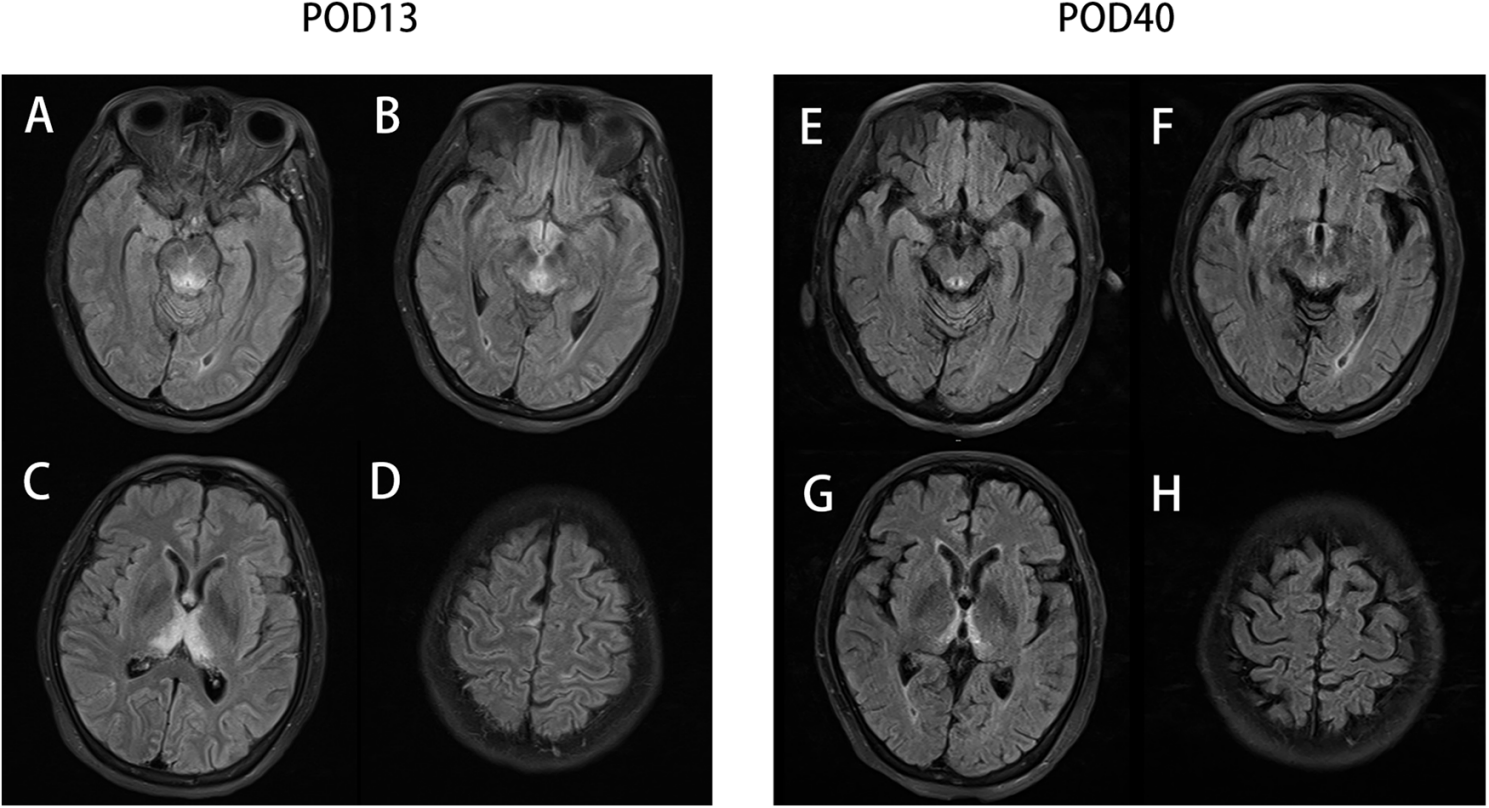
The T2-Flair sequencing MR image of the periaqueductal region of the midbrain (A), mammillary bodies (B), thalamus (C), and parietal cortices (D) on postoperative day 13 (POD13) and the T2-Flair sequencing MR image of the periaqueductal region of the midbrain (E), mammillary bodies (F), thalamus (G), and parietal cortices (H) on POD 40.
